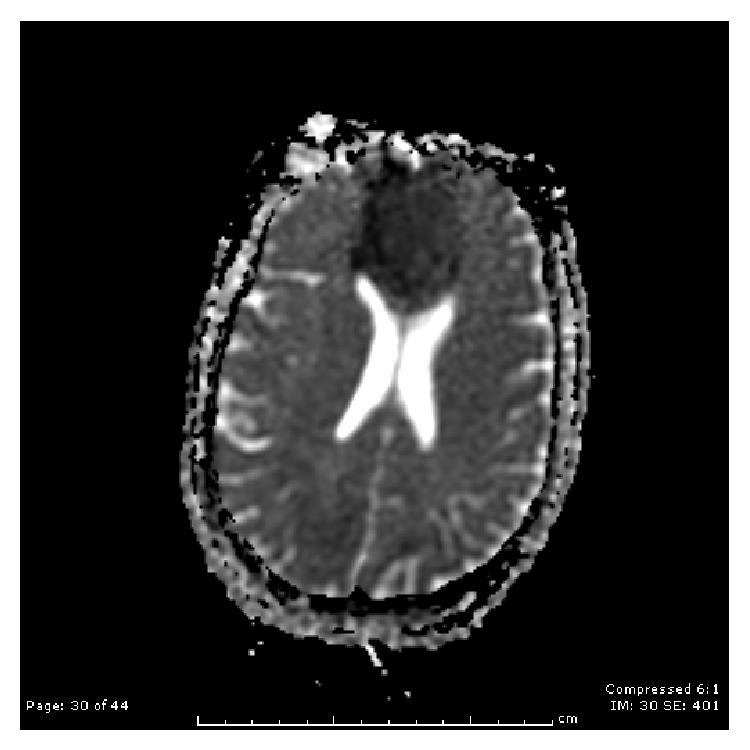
ADC MRI showing mismatch bilateral ACA territory.
